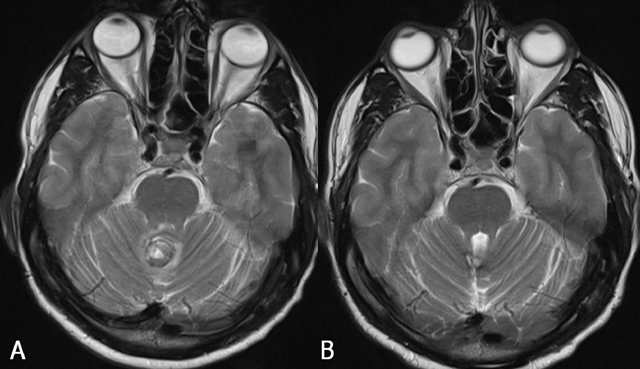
Resection of a cavernoma: pre- and postoperative MRI. Axial TSE T2-WI. A large cavernoma (A) is present in the vermis of the cerebellum. After resection (B), there is only a minimal loss of brain parenchyma and a small marginal region with susceptibility artifacts.
